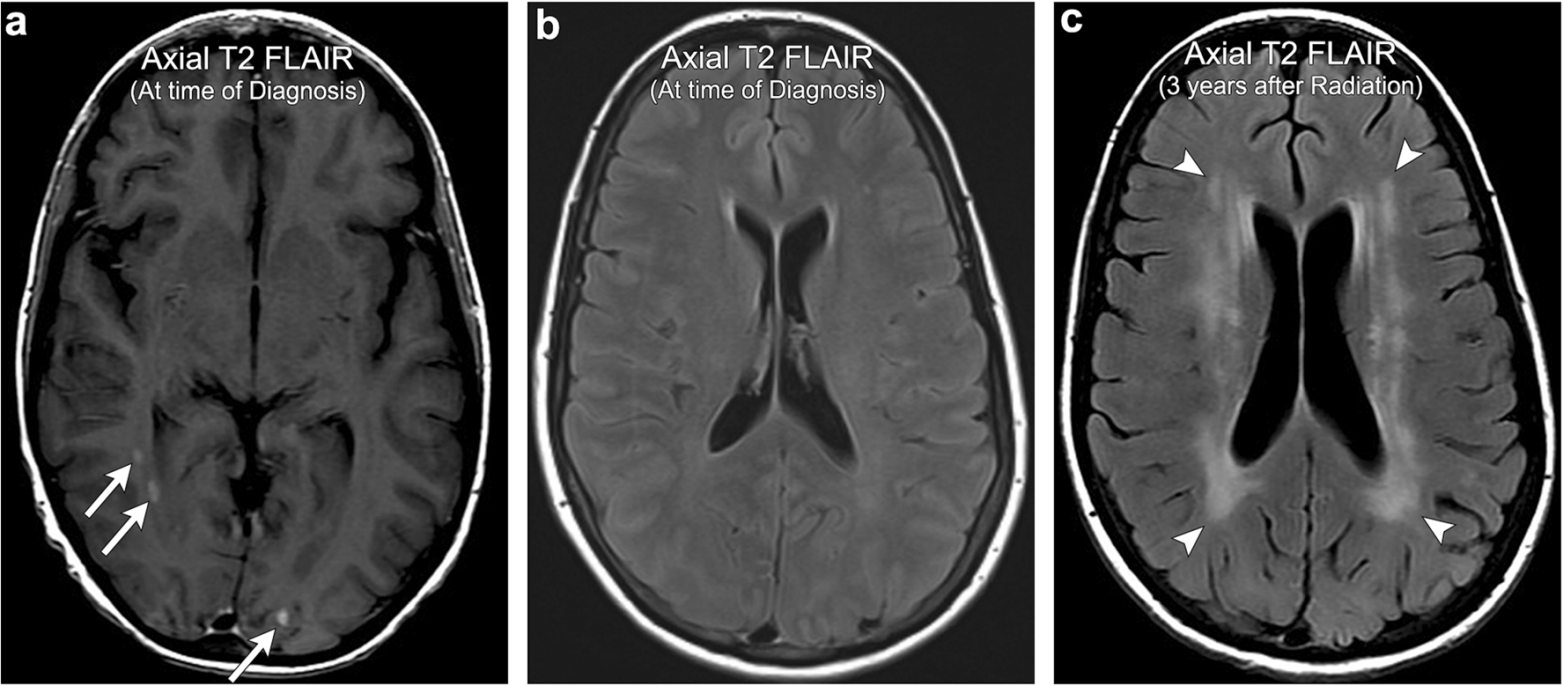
Radiation-induced leukoencephalopathy. A 47-year-old female with a history of multiple intracranial thyroid carcinoma metastases (arrows). The patient received WBRT and then developed gradual cognitive decline over the subsequent few years. Imaging performed 3 years after WBRT demonstrates extensive periventricular T2 FLAIR hyperintensity (arrowheads), more than would be expected for age-related microvascular disease, consistent with radiation-induced leukoencephalopathy. Note the relative sparing of subcortical U fibres
